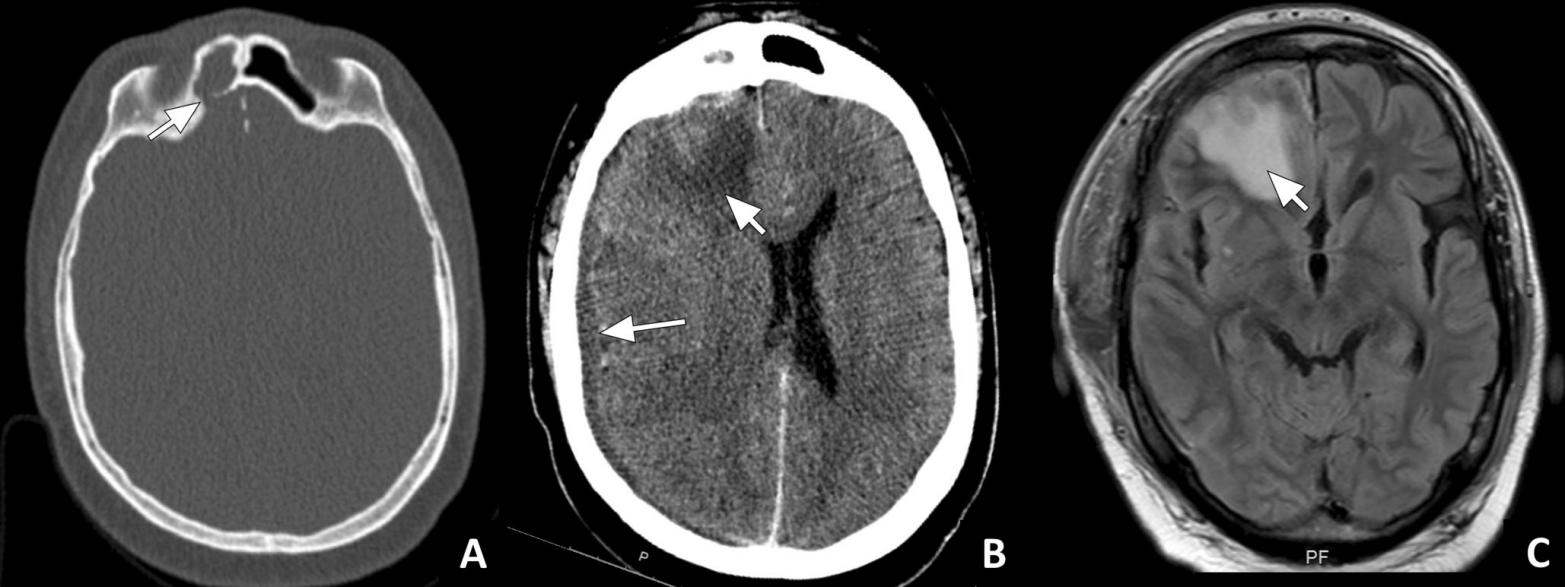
Focal cerebritis: a) Axial CT in bone window showing an opacified right frontal sinus with a bony defect in the posterior sinus wall (arrow). b) ceCT image in a soft tissue window in the same patient showing a focal area of hypodensity in the right frontal lobe (short arrow) with faint enhancement in the centre. In addition, a subdural fluid collection can be seen (long arrow). c) Axial MR FLAIR image of the same patient showing an area of hyperintensity in the left frontal lobe (short arrow). These findings indicate frontal sinusitis with bony erosion causing subdural empyema and focal cerebritis
